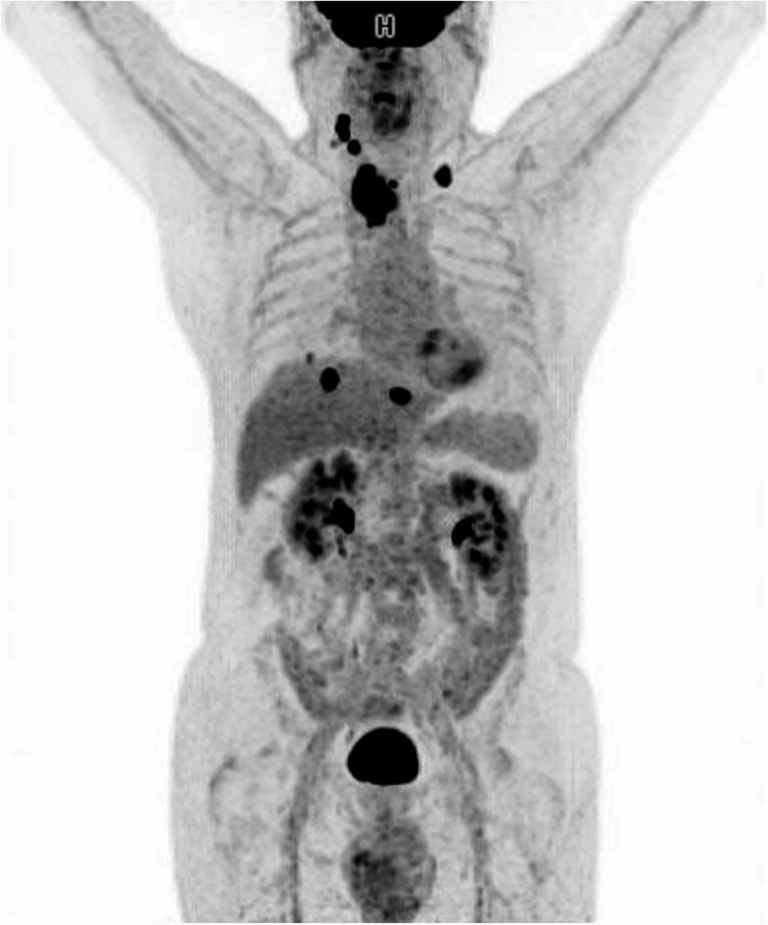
Initial 18F-FDG PET-CT. Positron emission tomography with 18F-fluorodeoxyglucose (18F-FDG PET/CT) at diagnosis revealed a primary lesion in the right thyroid lobe with tracheal extension (SUVmax: 9.30), along with bilateral lymph node involvement: right lateral cervical nodes (levels IIA and IIB, SUVmax: 4.53) and left retroclavicular node (SUVmax: 4.03). Additionally, multiple hypermetabolic hepatic foci suggestive of metastatic spread were identified, including one intrahepatic lesion in segment VIII (SUVmax: 6.24) and two peripheral lesions in segments II and VIII (SUVmax: 4.84 and 2.30, respectively)
